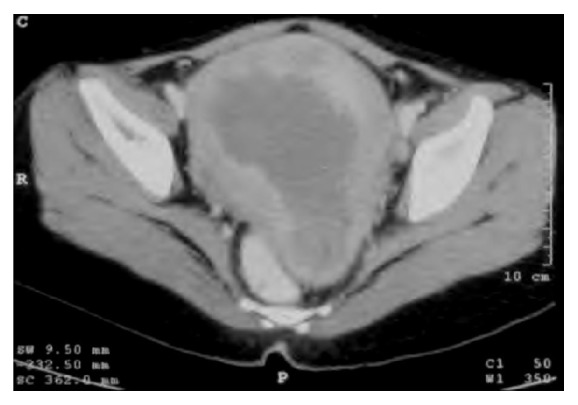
Computed tomography of the abdominopelvic region shows an enlarged well-defined border uterus with diffused enhancing thickening in the endometrial cavity.
